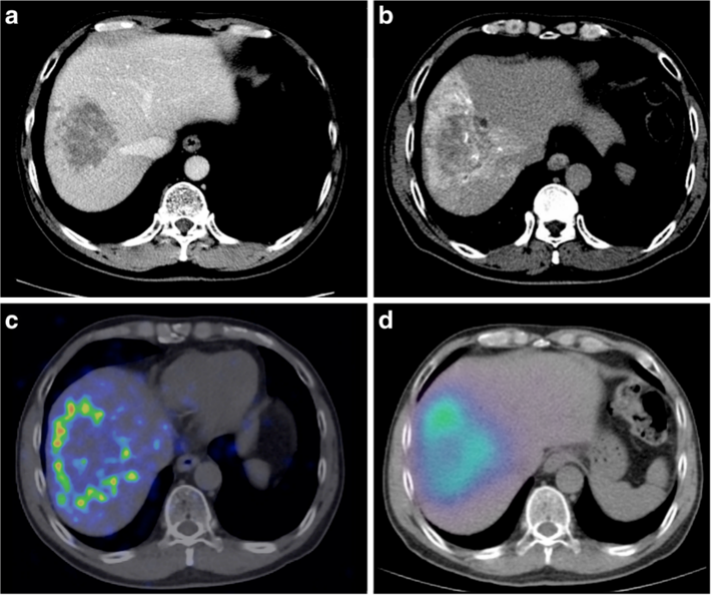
Patient 20. (a) Large hypovascular HCC in the right lobe, seen on contrast-enhanced CT Abdomen. (b) Catheter-directed CT angiogram of the right hepatic artery demonstrates tumor hypovascularity and delineates the target arterial territory. (c)^90^Y PET/CT depicts, in high resolution, a rim-like activity in the tumor periphery with a large area of relative central photopenia, typical of hypovascular tumors. (d)^90^Y bremsstrahlung SPECT/CT poorly demonstrates the peripheral activity due to its low image resolution and under-represents the extent of central photopenia.
